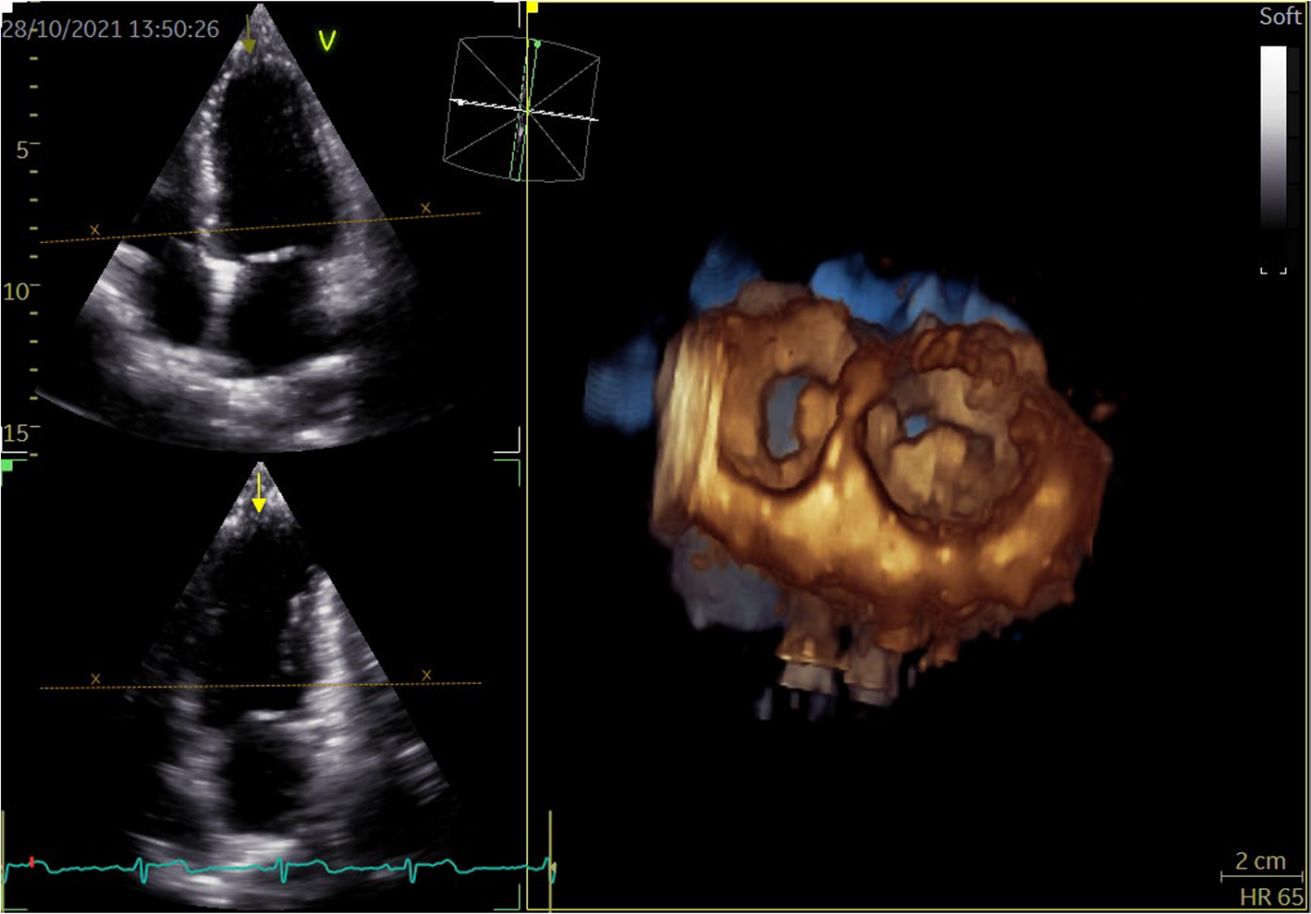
LA and LV full-volume images were obtained in apical four-chamber views, and high-quality 3D images of 4–6 cardiac cycles were recorded during sinus rhythm. LA, left atrial; LV, left ventricular; 3D, three-dimensional
